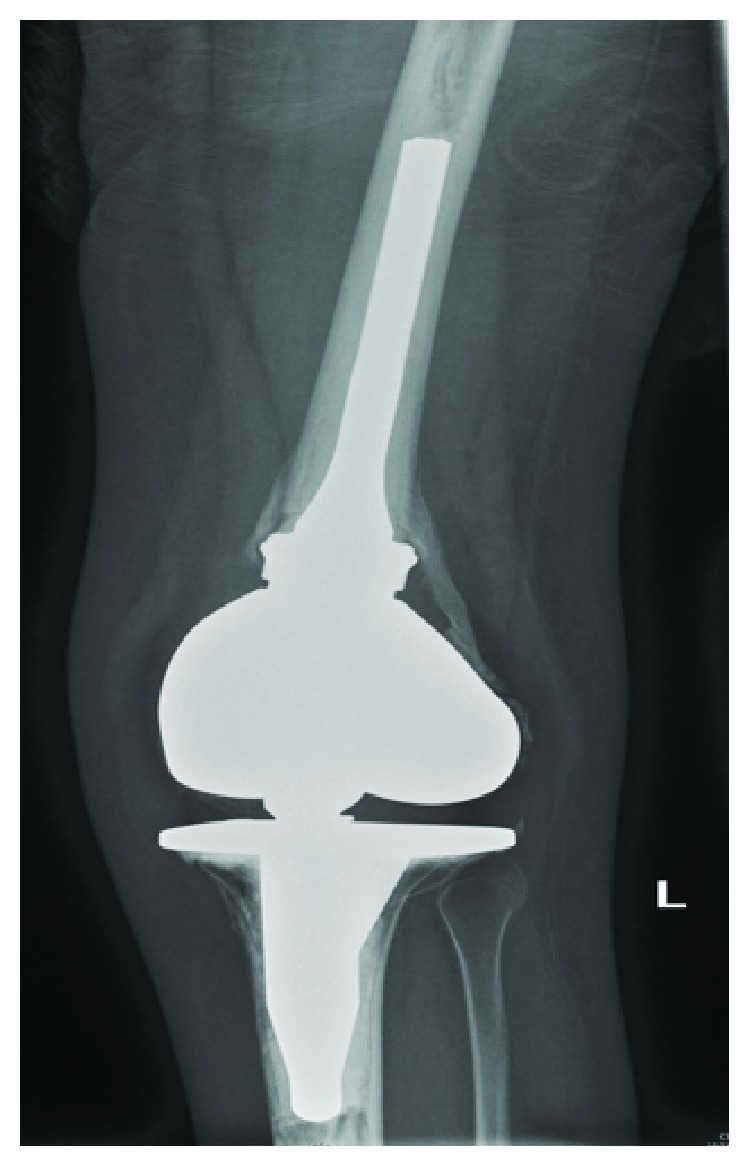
Postoperative plain radiograph of constrained left knee replacement.
